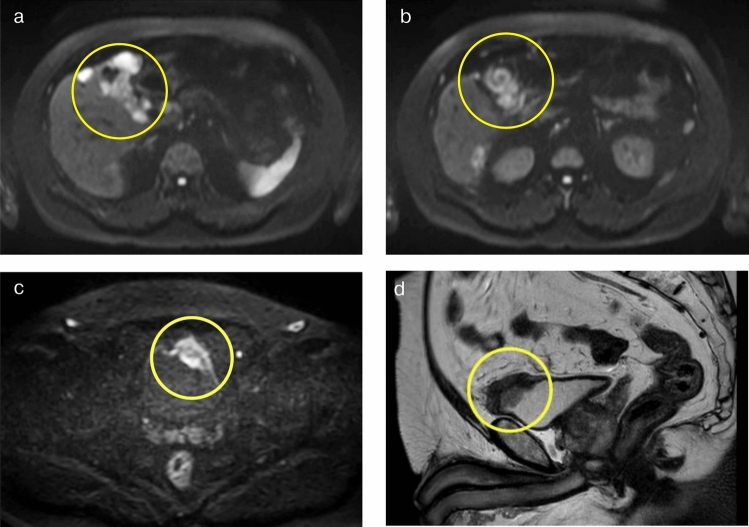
MRI findings. a ~ c Diffusion weighted images: a. b. Diffusion restriction in the gallbladder wall and adjacent liver. c Diffusion restriction in the urinary bladder mass with irregular wall thickening. d T2-weighted image. The mass was located at upper ventral side of the urinary bladder in the sagittal view
